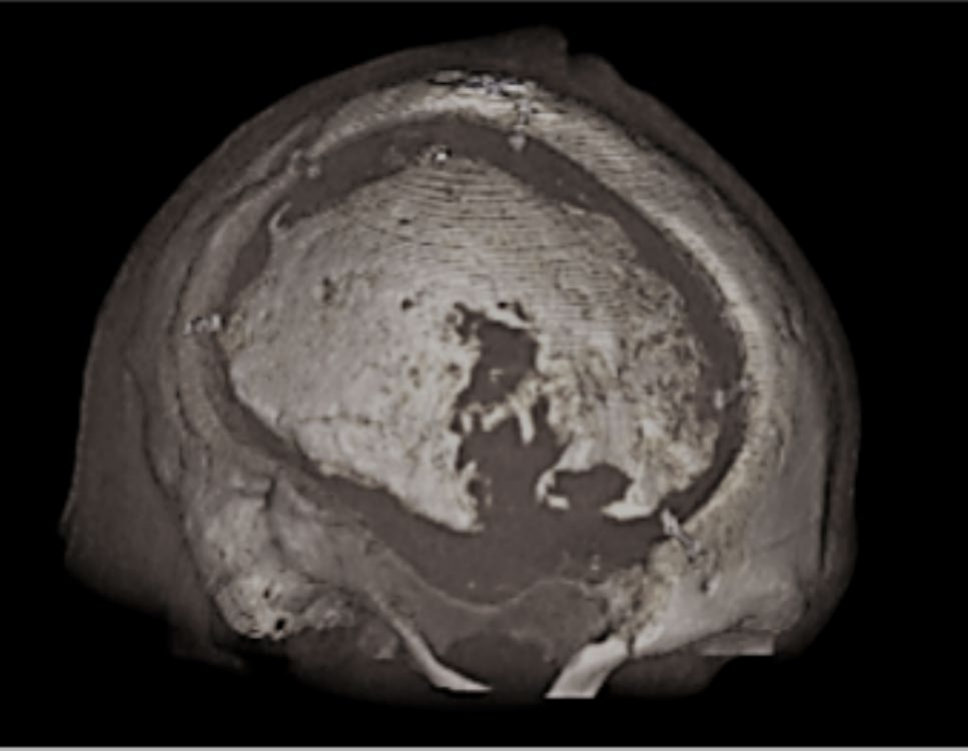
Computed tomography of aseptic bone flap necrosis (ABFN). Example case of aseptic bone flap necrosis; 3D reconstruction. Observed in a 51-year-old man who had undergone a right-sided hemicraniectomy due to an acute subdural hematoma and subsequent reimplantation of an autologous bone flap
